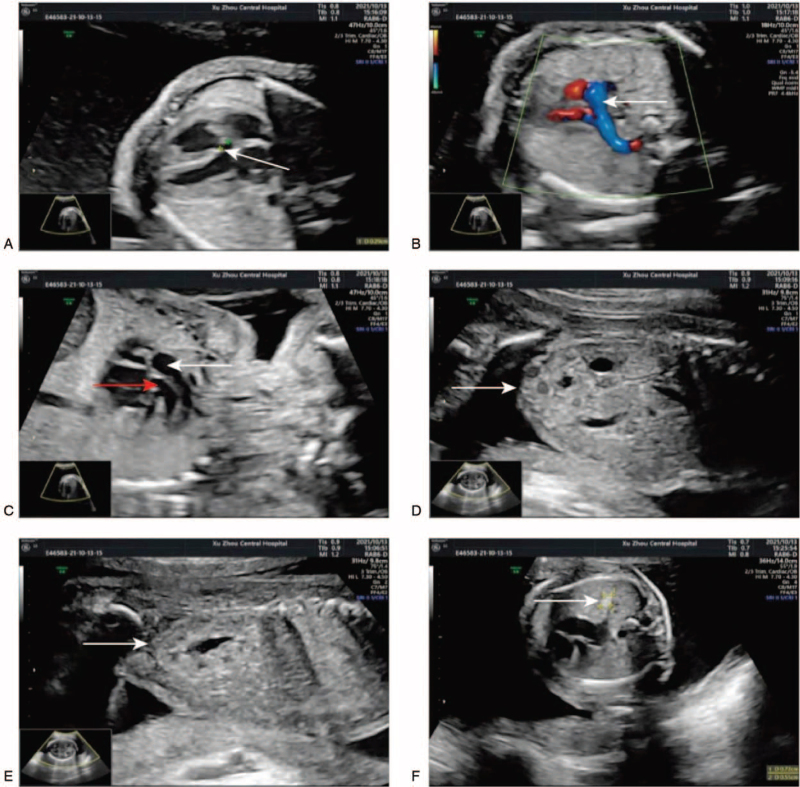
Prenatal ultrasound results: (A) ventricular septal defect, (B) aorta predominantly from the right ventricle, (C) the pulmonary artery (red arrow) originates in the right ventricle and runs parallel to the aorta (white arrow), (D) multiple cysts of the right kidney, (E) hyperechogenic kidneys, and (F) hyperechoic mass in the right lung.
